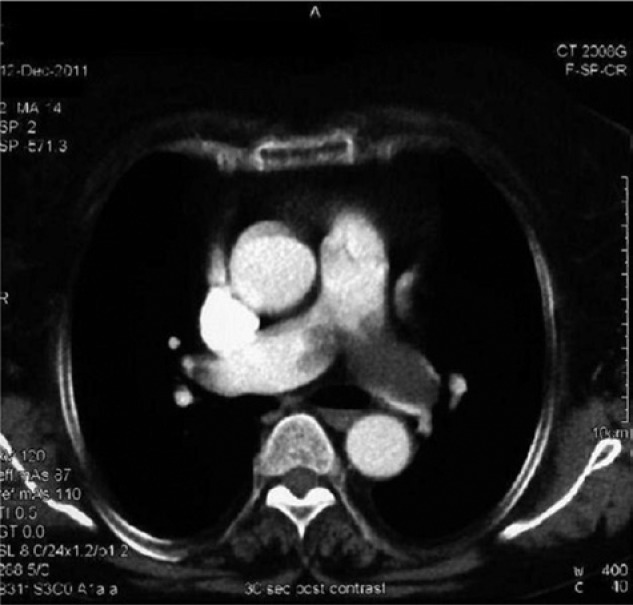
Computed tomography scan of chest showing saddle-shaped filling defect in the main
